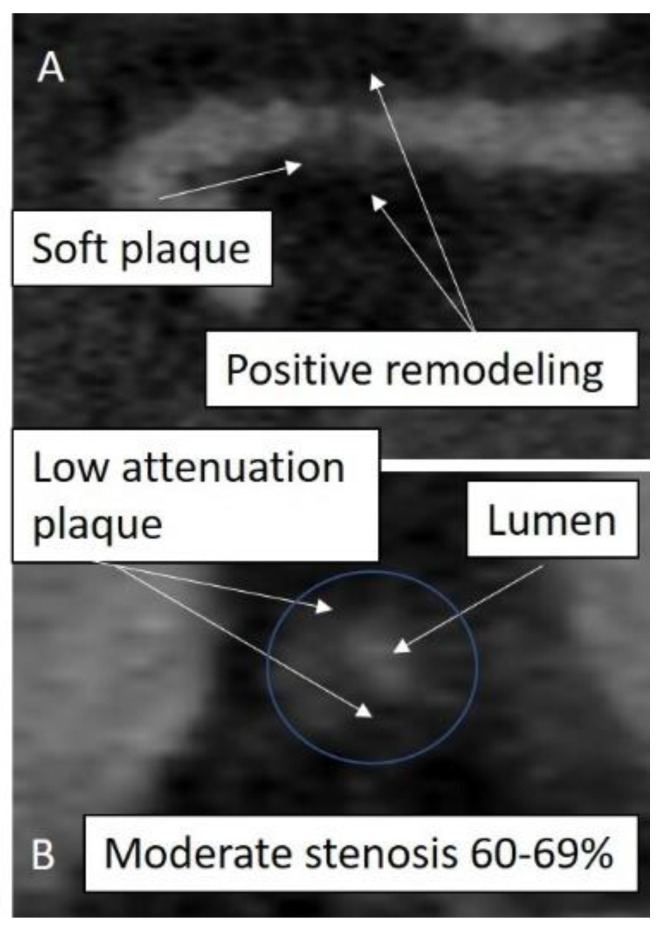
Subclinical atherosclerosis. Coronary CT angiogram (CCTA) of a patient undergoing cardiac CT prior to pulmonary vein ablation therapy. CAC was zero. A subclinical 50% stenosis was detected in the proximal left anterior descending artery (LAD) with features suggestive of an unstable plaque. Multiplanar reformatted images are shown in long axis (panel (A)) and short axis (panel (B)).
